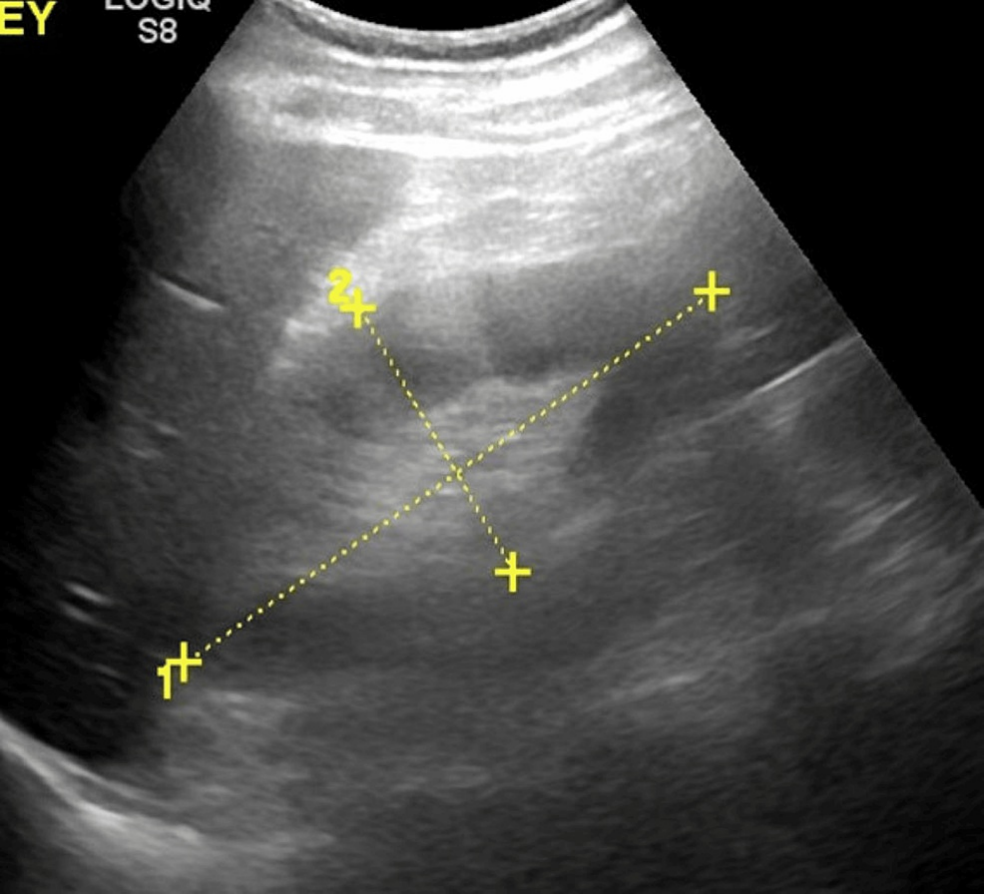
Ultrasound of the right kidney showing a normal kidney measuring approximately 12.6 x 5.9 x 5.3 cm. There were no cysts, solid mass lesions, hydronephrosis, or calculus, and cortical central differentiation was maintained in both kidneys.
